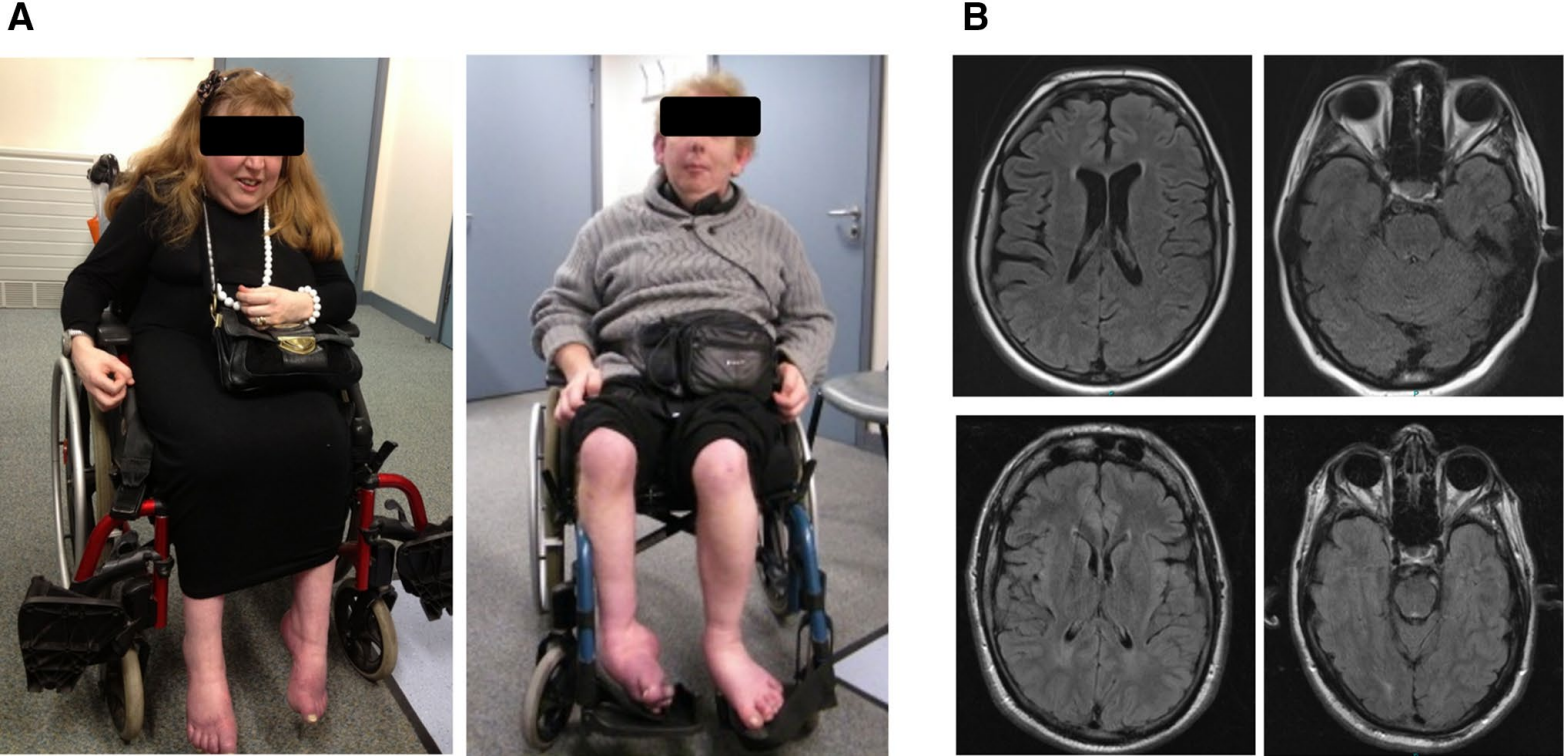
a P1 aged 40 years (left) and P2 aged 38 years. Both patients have characteristic facial features low-set ears and, and presented with distal upper and lower limb wasting and equinovarus deformity due to peripheral neuropathy and spastic paraparesis. b Axial T2 FLAIR MR images of P1 (top panels) and P2 (bottom panels). MRI brain in P1 aged 41 years showed mild global atrophy. MRI brain in P2 aged 34 demonstrated no significant abnormality apart from mild increased T2 signal in the peritrigonal white matter bilaterally
